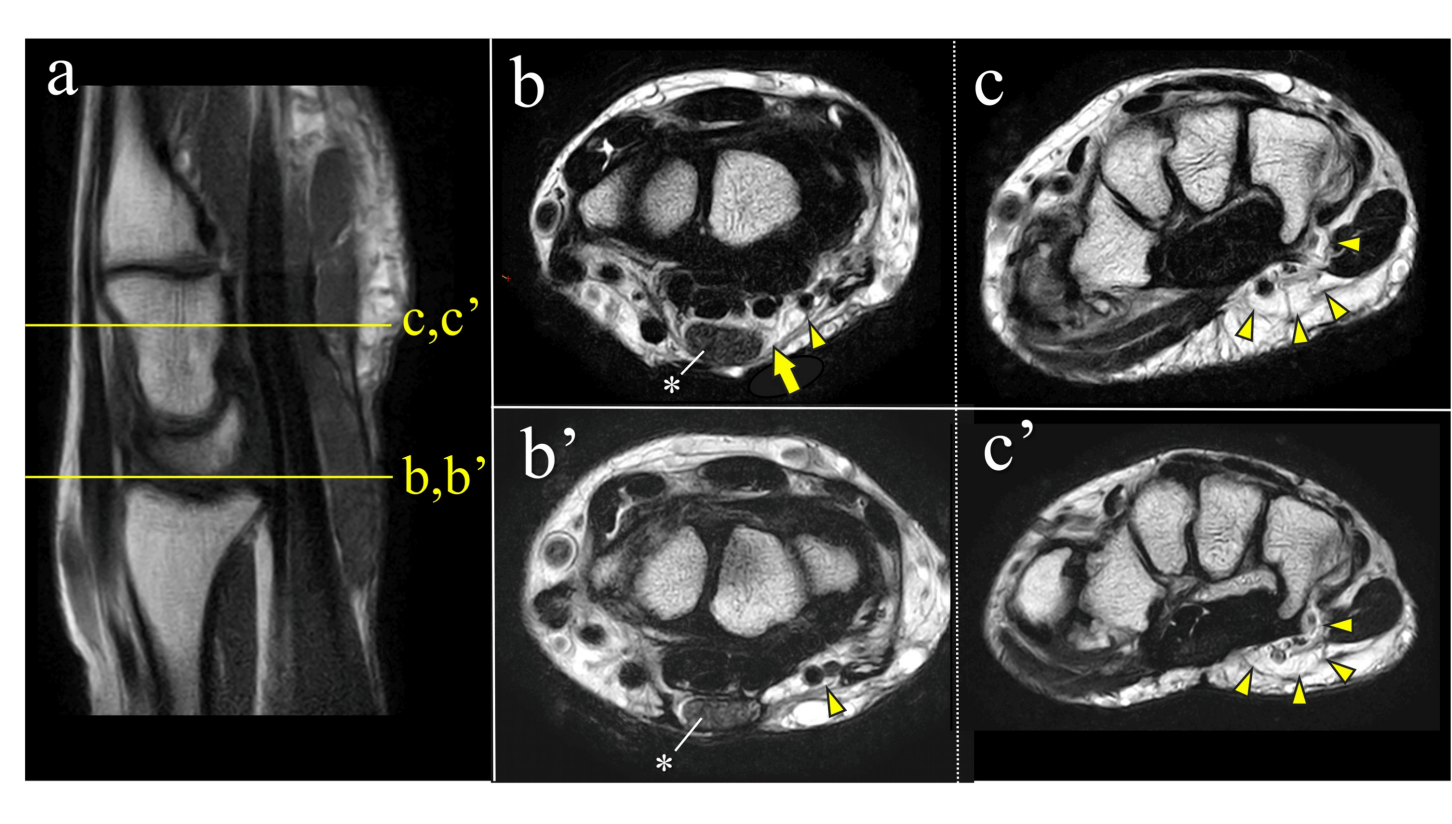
MRI findings of the lipoma at presurgery and POY1. (a) Scout view (T1-weighted sagittal image). (b, b′) T2-weighted sagittal images at the level of the proximal carpal and Guyon’s canals before surgery (b) and at POY1 (b′). The lipoma around the median nerve (b, yellow arrow) was excised and was no longer visible on the POY1 MRI (b′). The cross-sectional area of the median nerve decreased from 50 mm² preoperatively (b, *) to 35 mm² postoperatively (b’, *). A lipoma remained around the ulnar nerve proximally (b/b’, arrowhead). (c, c′) T2-weighted sagittal images distal to Guyon’s canal before surgery (c) and at POY1 (c′). A residual lipoma was evident distal to Guyon’s canal, around the ulnar nerve (yellow arrowheads). MRI, magnetic resonance imaging; POY, postoperative year.
